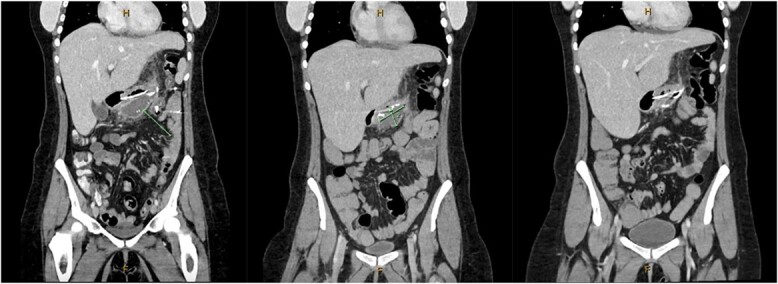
Coronal CT images on Days 2, 9 and 14 post insertion of nasobiliary drainage tube to sleeve gastrectomy staple line collection showing interval reduction in collection size.
